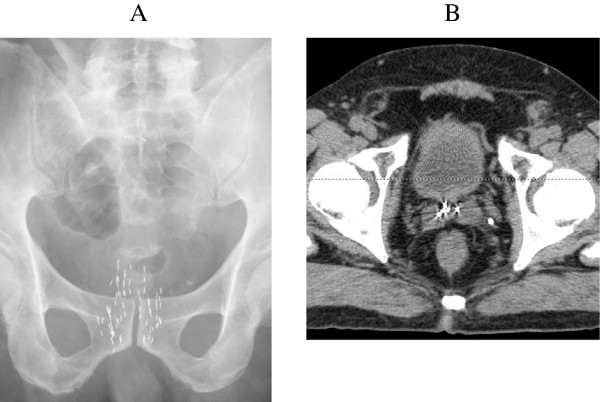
Pelvic X-ray and CT scan images after salvage brachytherapy. (A) Pelvic X-ray and (B) CT scan demonstrated the source at the bottom of seminal vesicle.
